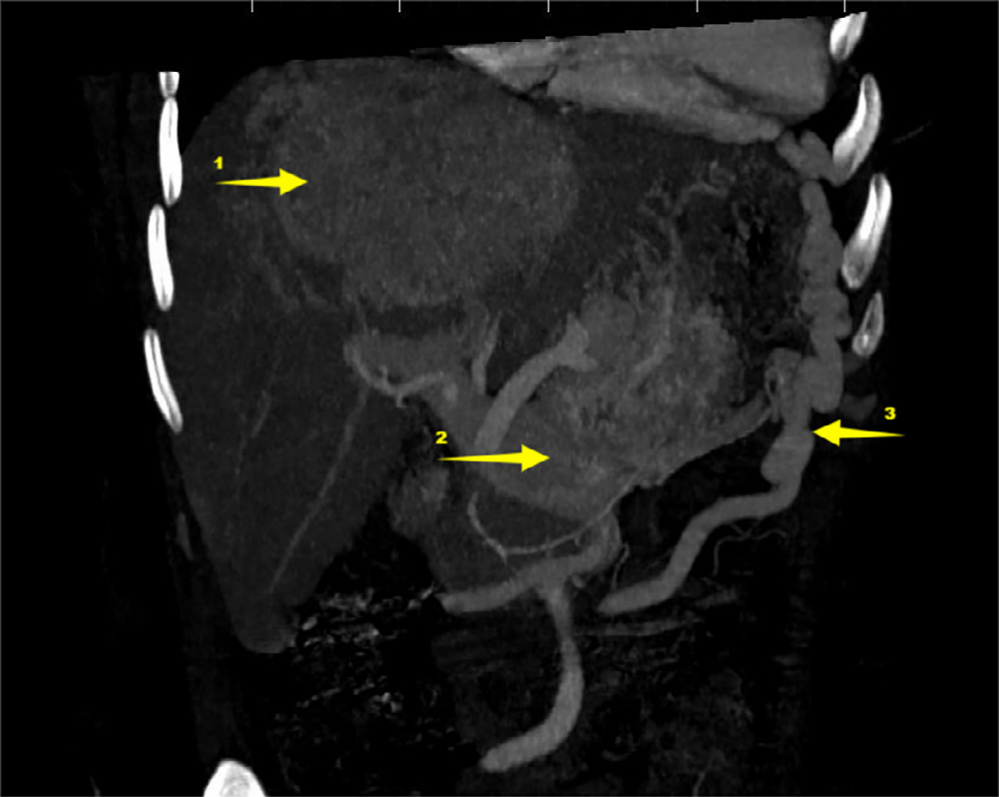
Abdominal injected CT on coronal plane with maximum intensity projection mode 1 liver tumor. 2 tumoral thrombosis inside portal vein 3 collateral circulation.
